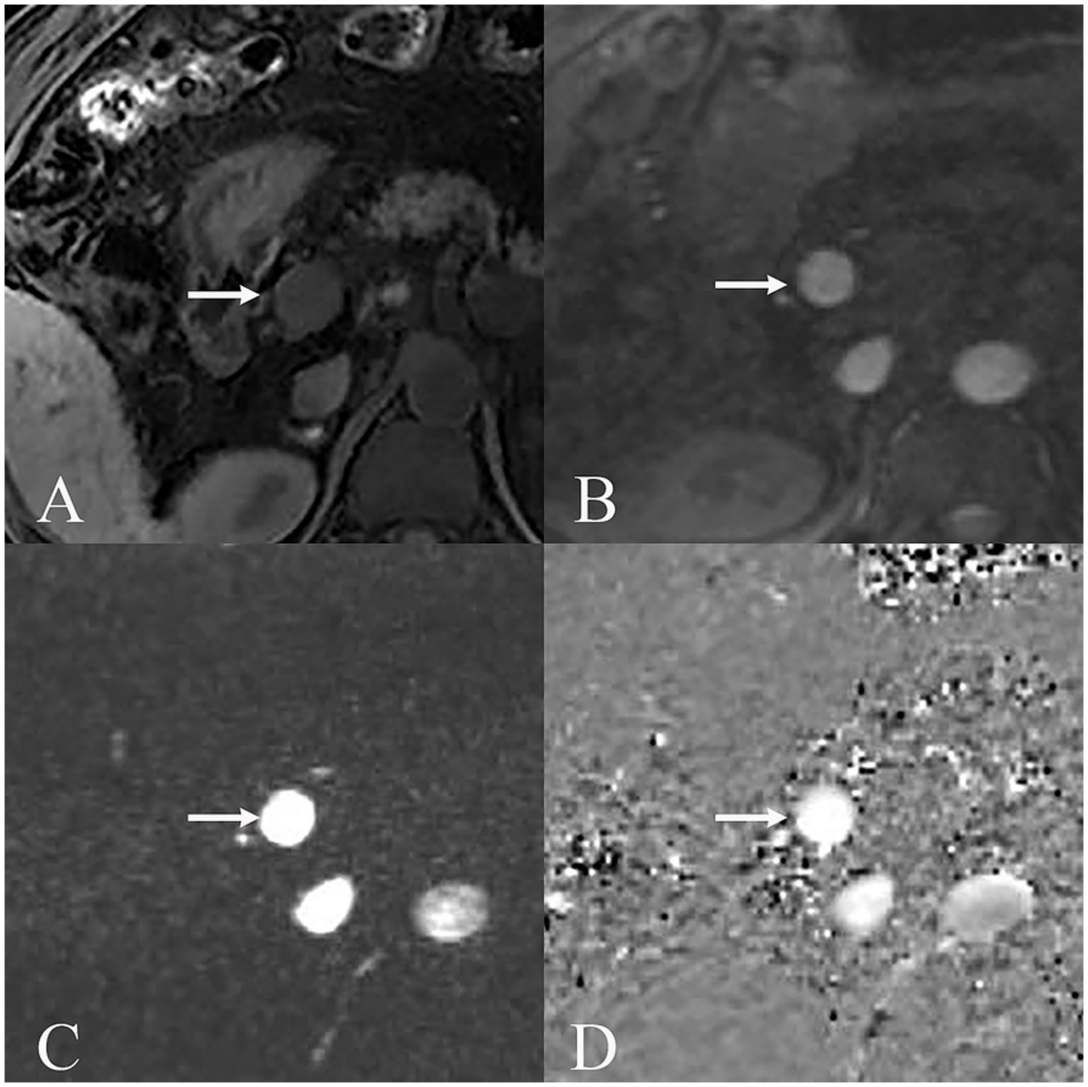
MRI images displaying portal vein (arrow) under various sequences used for MRI 4D Flow. (A) Non-enhanced T1WI showed the main portal vein and surrounding anatomical structures. (B) Scout image displays the portal vein. (C) Magnitude image demonstrates the portal vein with good vessel contrast and definition for flow quantification. (D) Phase contrast image shows flow-related signal intensity changes within the portal vein, where signal intensity corresponds to velocity encoding in the through-plane direction.
